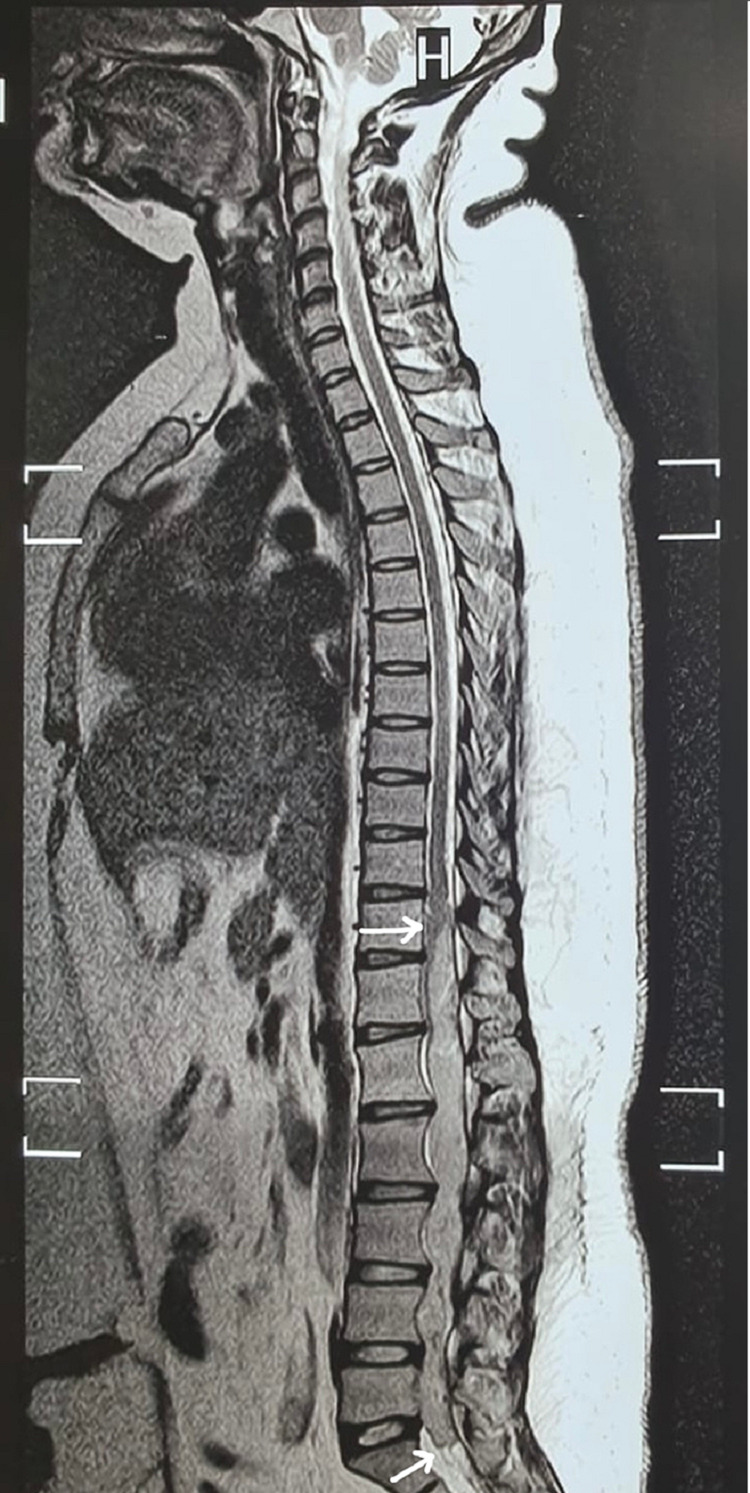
Sagittal T2-weighted image of the spine showing a space-occupying lesion extending from the D11 to S1 segments
